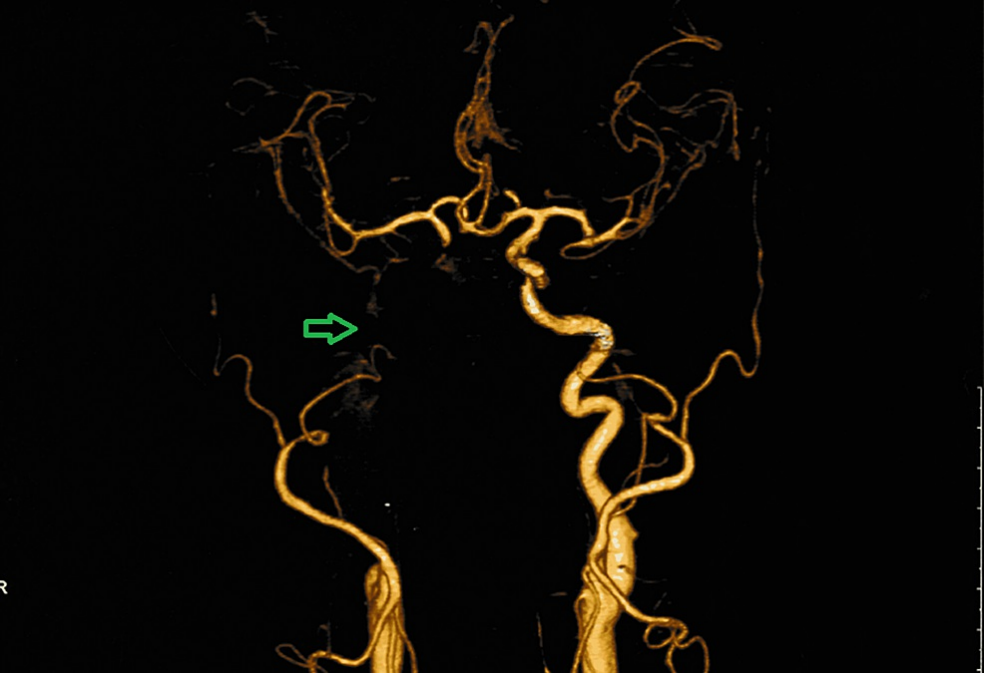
CT angiography cerebral and neck vessels showing non-visualized or completely occluded right internal carotid artery (green arrow) from its origin carotid bifurcation to supraclenoid part and a good collateral system. CT: computerized tomography.
